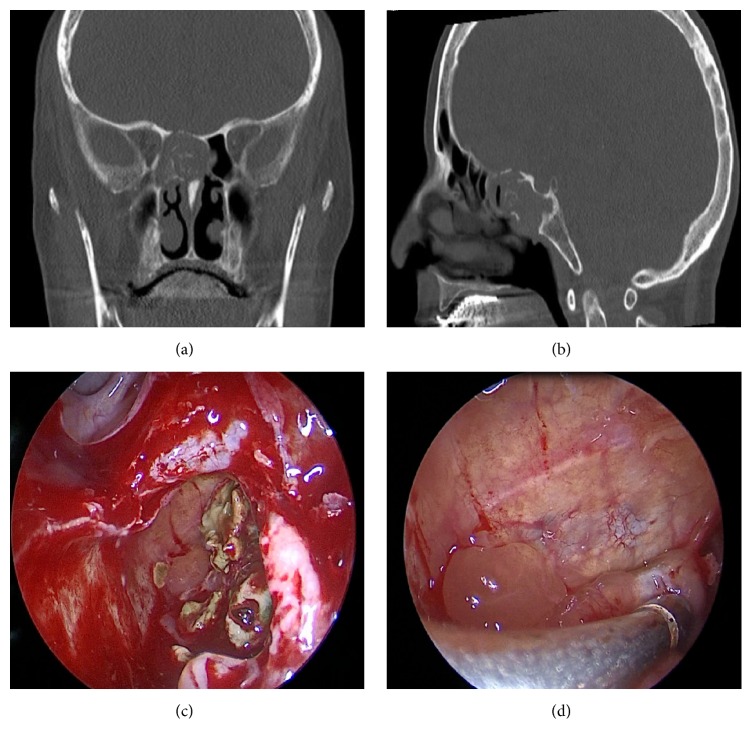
(a, b) Paranasal sinus computed tomography of a soft tissue mass filling the right posterior ethmoidal cells and sphenoid sinus. (c) Endoscopic view of the yellow-green colored caseous material in the right sphenoid sinus. (d) Endoscopic view of the intact dura mater in the right sphenoid sinus.
